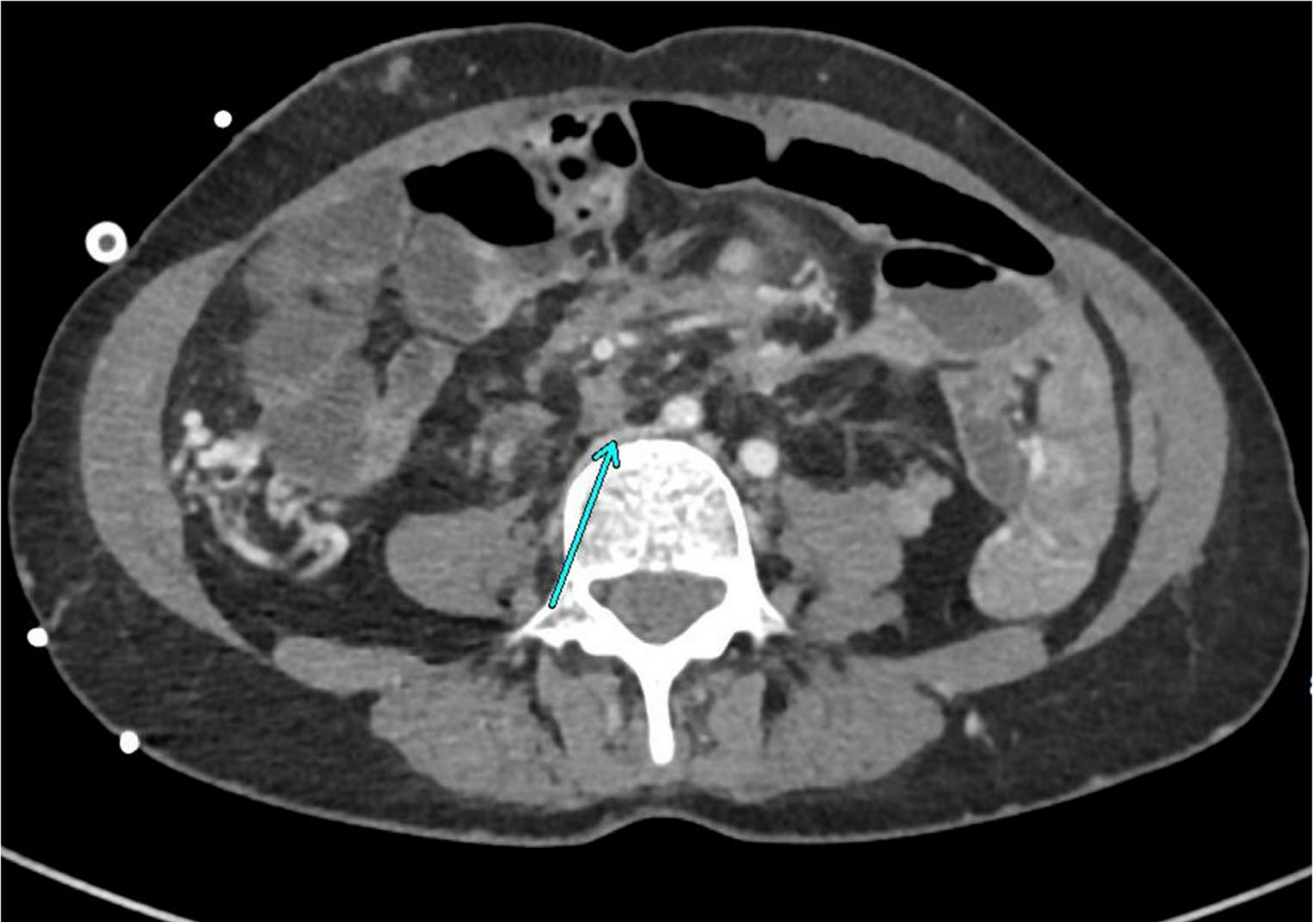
CT axial image: arrow indicates desmoid compressing iliac/inferior vena cava confluence
